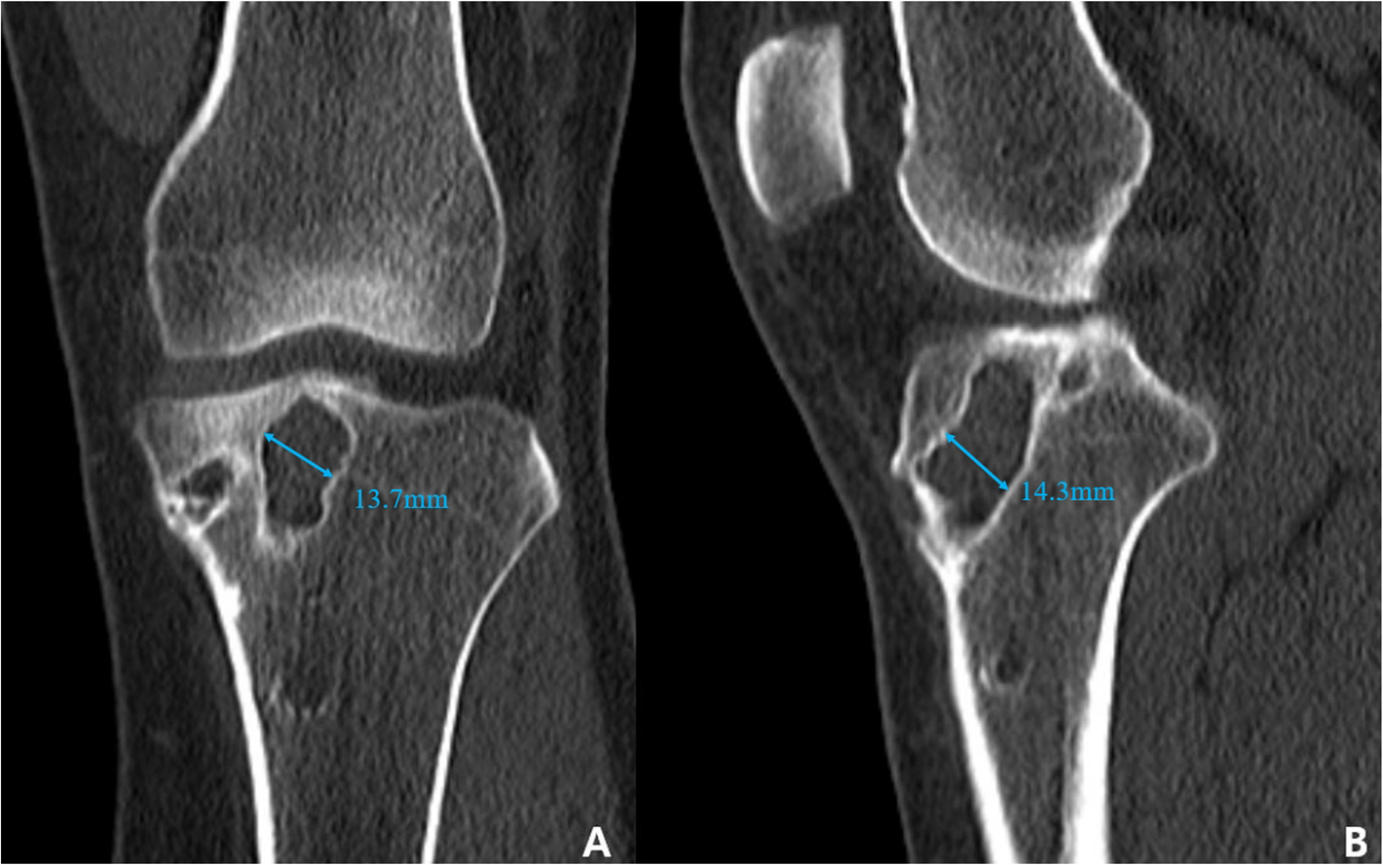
Coronal (a) and sagittal (b) view of computed tomography (CT) images demonstrate widening of the tibial tunnel in the setting of a failed anterior cruciate ligament reconstruction. Measurements are made perpendicular to the axial plane of the tunnel at the widest point
